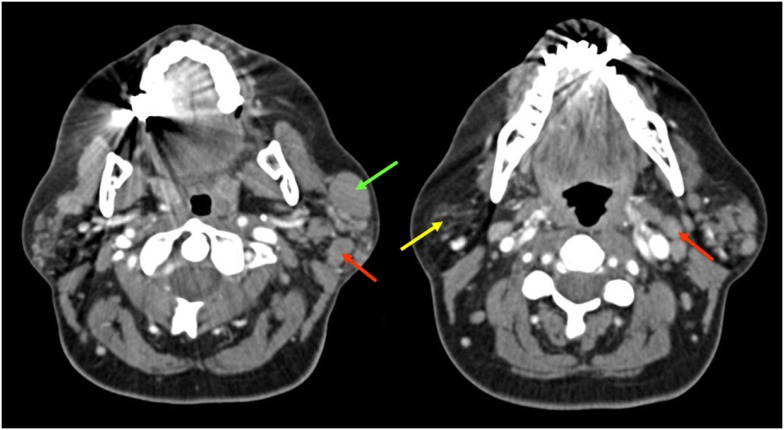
Contrast-enhanced computed tomography demonstrated fatty atrophy of the parotid glands (yellow arrow), enhancing predominately left parotid nodule (green arrow), and enlarged cervical lymph nodes (red arrows).
